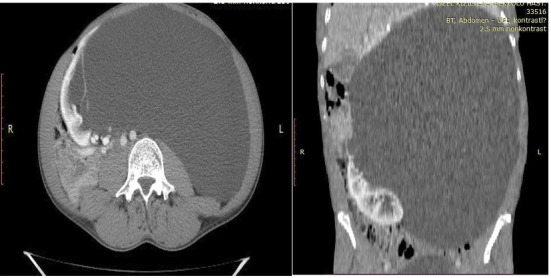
Computed tomography scan of our patient. Sagittal and coronal views of computed tomography of the abdomen and pelvis demonstrate a large cyst arising from the interpolar region of the left kidney. The cyst measures 40 × 28 cm in craniocaudal and transverse dimensions, respectively.
